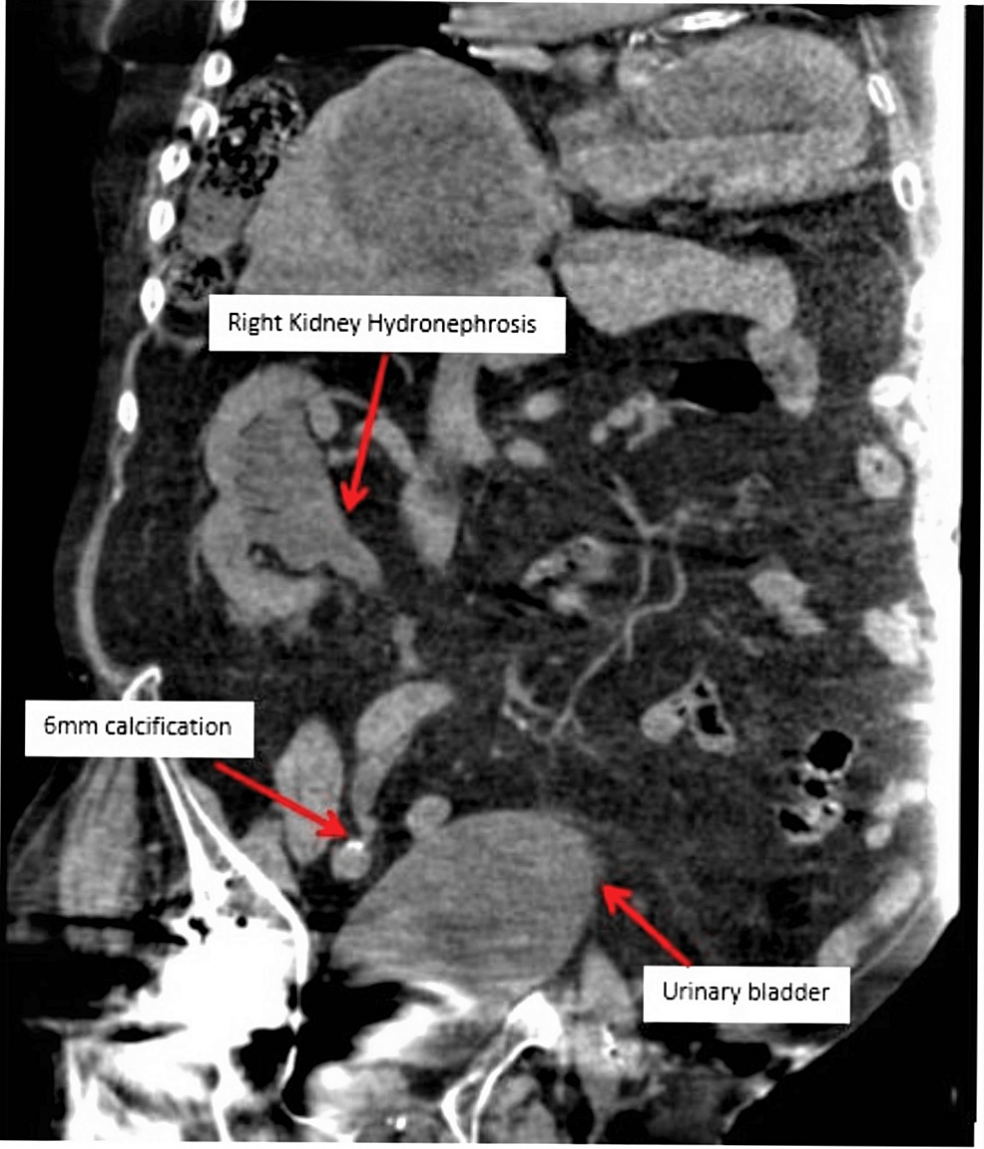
Abdominal CT demonstrating right kidney hydronephrosis and distal 6 mm calcification
